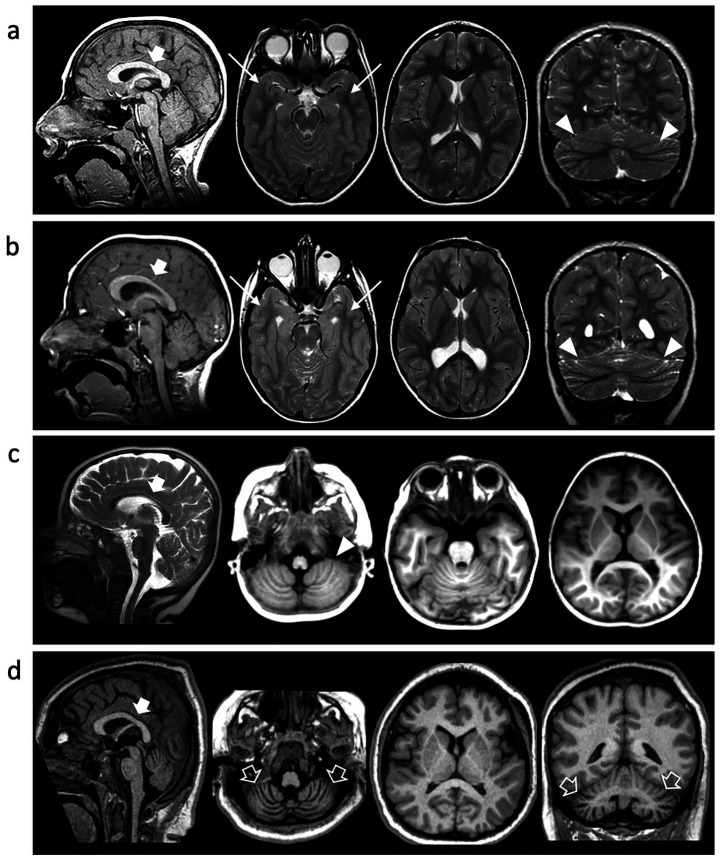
Neuroimaging findings. Brain MRI of Individual 1 at 10y (A), Individual 2 at 7 y (B) and Individual 4 at 2 y (C) and 10y (D). Sagittal T1 or T2-weighted images (first column), axial images (second and third columns), and coronal images (last column). Microcephaly with disproportionally large corpus callosum (thick arrows), simplified gyral pattern and cerebellar foliar anomalies are noted in all cases (arrowheads). There is bilateral temporo-basal dysgyria in Individual 1 and Individual 2 (arrows). Progressive cerebellar atrophy is detected in Individual 3 over the course of 8 years (empty arrows).
